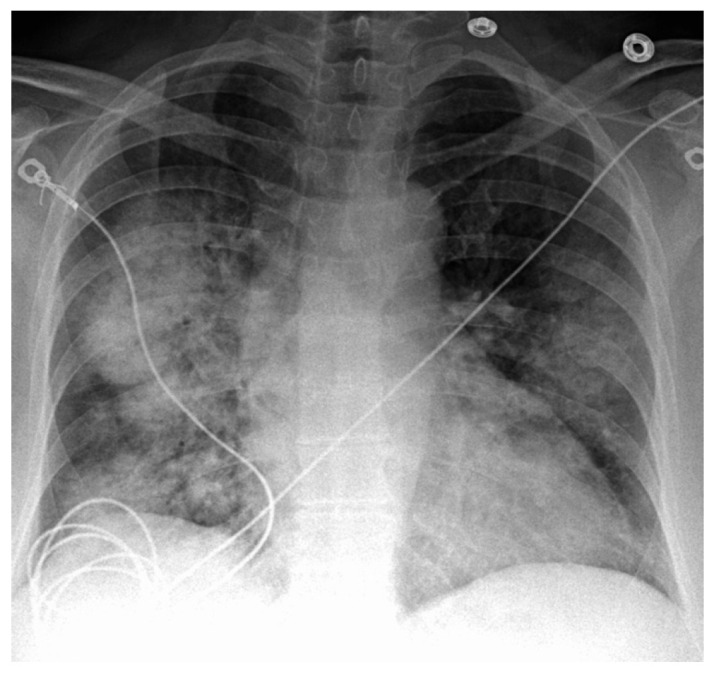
Chest X-ray showing asymmetric, diffuse, bilateral heterogenous airspace disease and consolidations in a patient with SLE, consistent with DAH. (This work has been reproduced from Jamsheer et al., 2024, in accordance with the terms of the Creative Commons Attribution License [32]).
